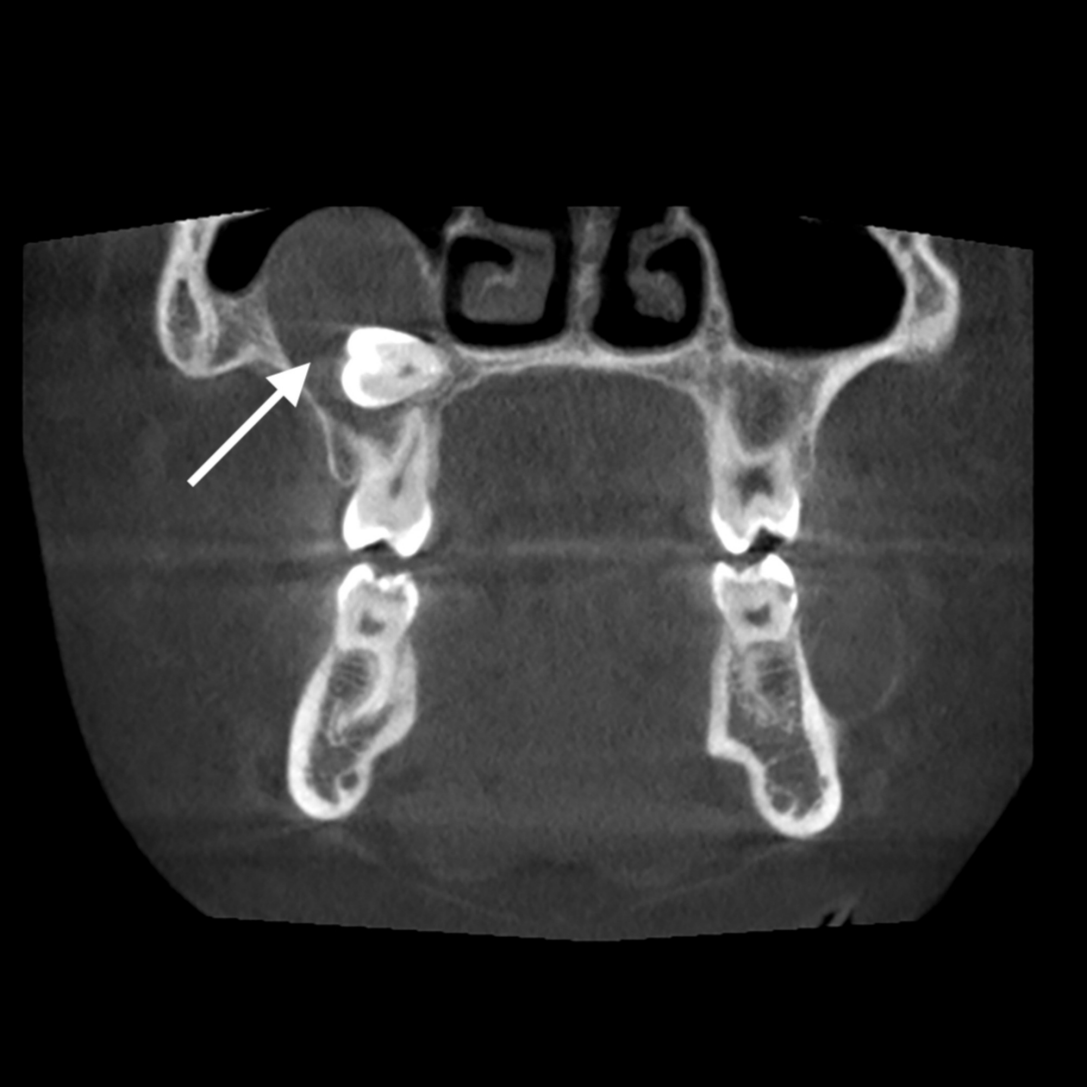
Cone beam computed tomography (CBCT) of another large lesion in the maxilla. Cone beam computed tomography (CBCT) revealed another large lesion associated with the impacted right maxillary second molar, which extended up to the right maxillary sinus (white arrow).
